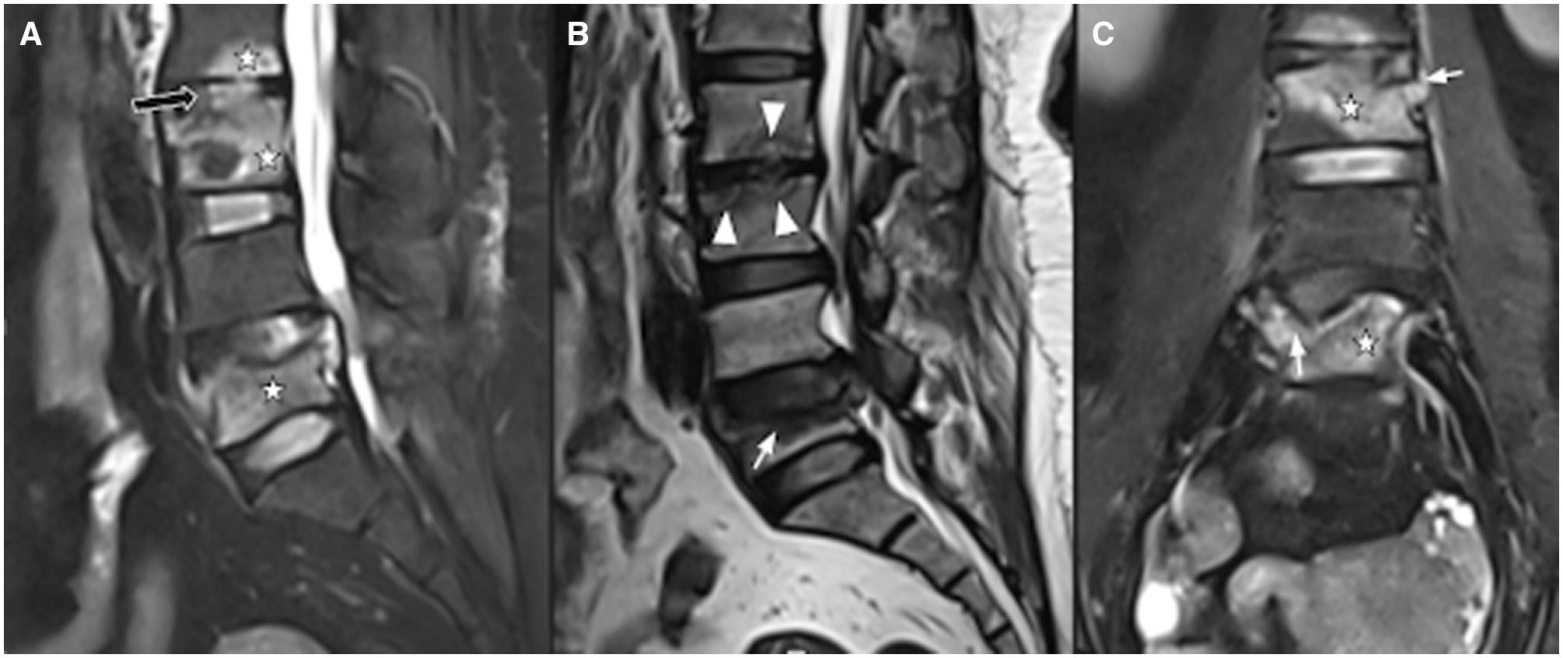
MRI of lumbar spine shows subchondral fractures in endplates of L2, L3, and L5 vertebra with collapse and marrow oedema, intradiscal fluid signal and double line sign—marrow oedema in the body of L2, L3, and L5 vertebrae (star), collapse with reduced height of L3 vertebra and near complete collapse of right lateral half of body of L5 vertebra (white arrow) and intradiscal fluid signal (black arrow) on sagittal and coronal STIR images (A) and (C). Subchondral fracture in L2, L3, and L5 vertebral body and double line sign in endplates of L2 and L3 vertebra (white arrowheads) on T2W image (B).
